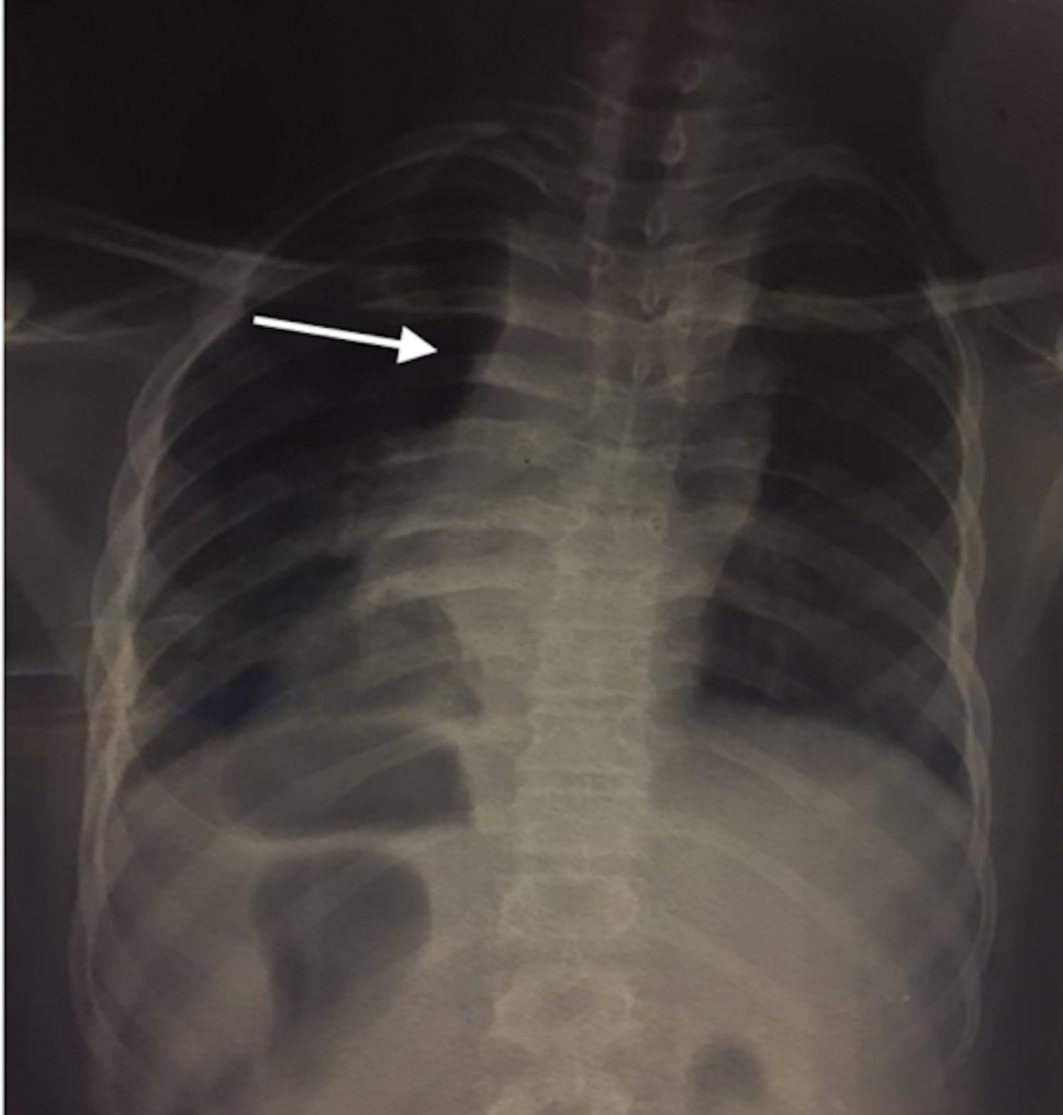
Chest X-ray Showing mediastinal widening.
